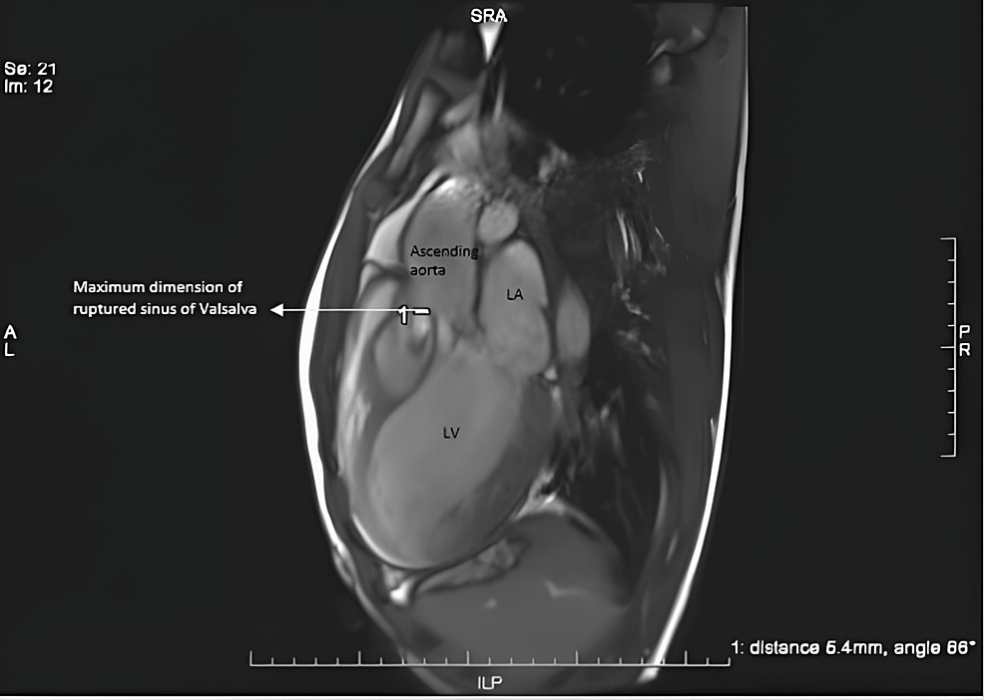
Cardiac MRI represents the dimension of a ruptured opening in the right sinus.
